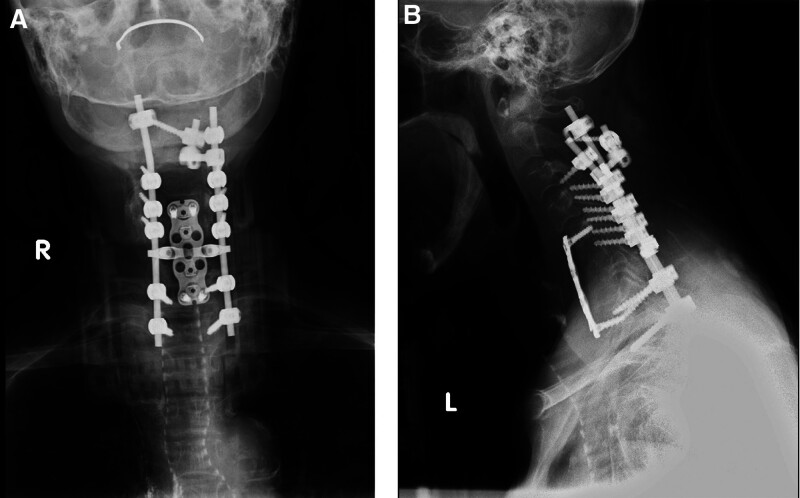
Cervical spine X-ray after posterior operation performed. (A) The anteroposterior view; (B) the lateral view.
